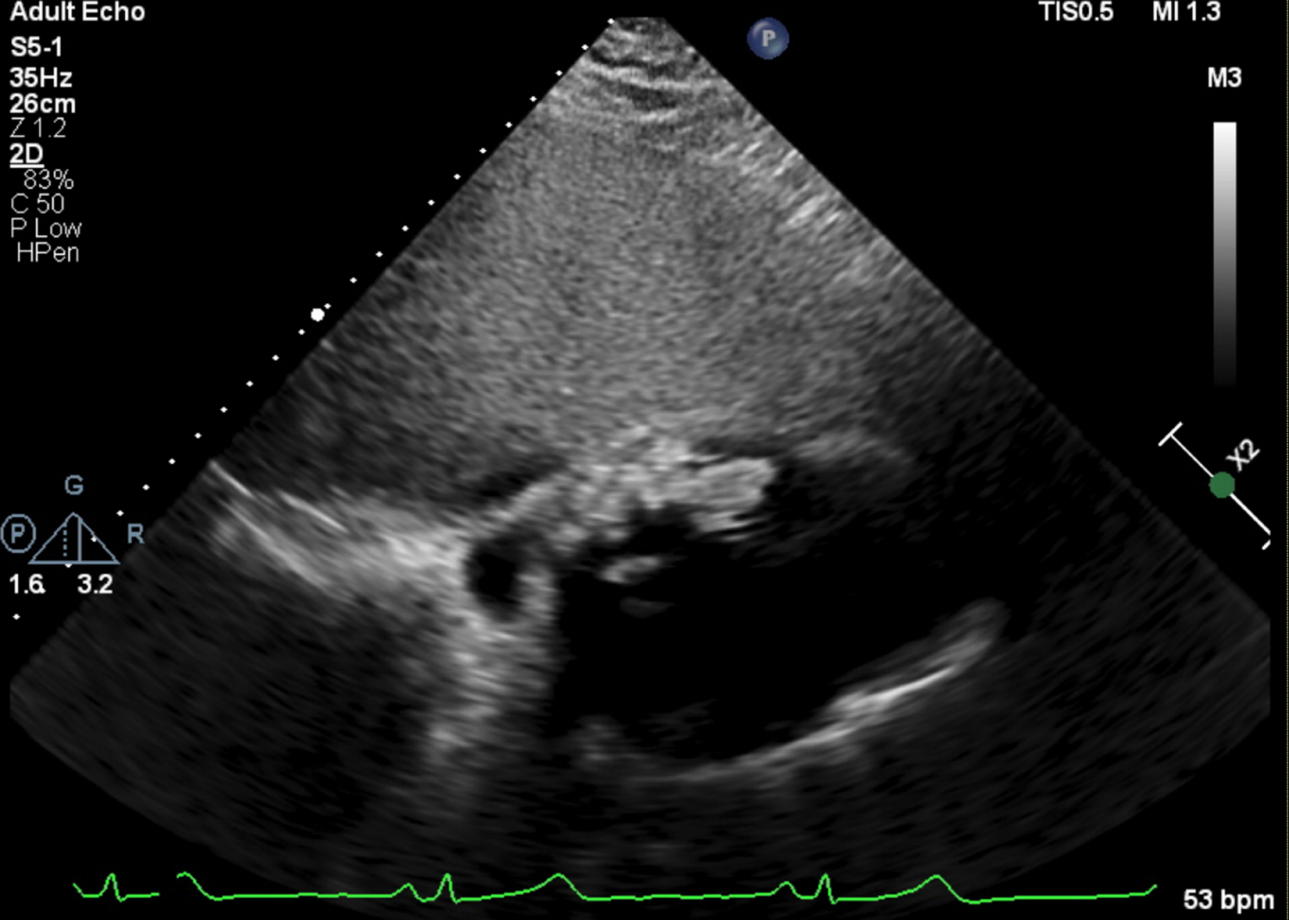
Transthoracic echocardiogram during evaluation in the emergency department confirmed the presence of a small pericardial effusion and showed significant clot burden and possible intracavitary right ventricular mass which was incompletely characterized.
